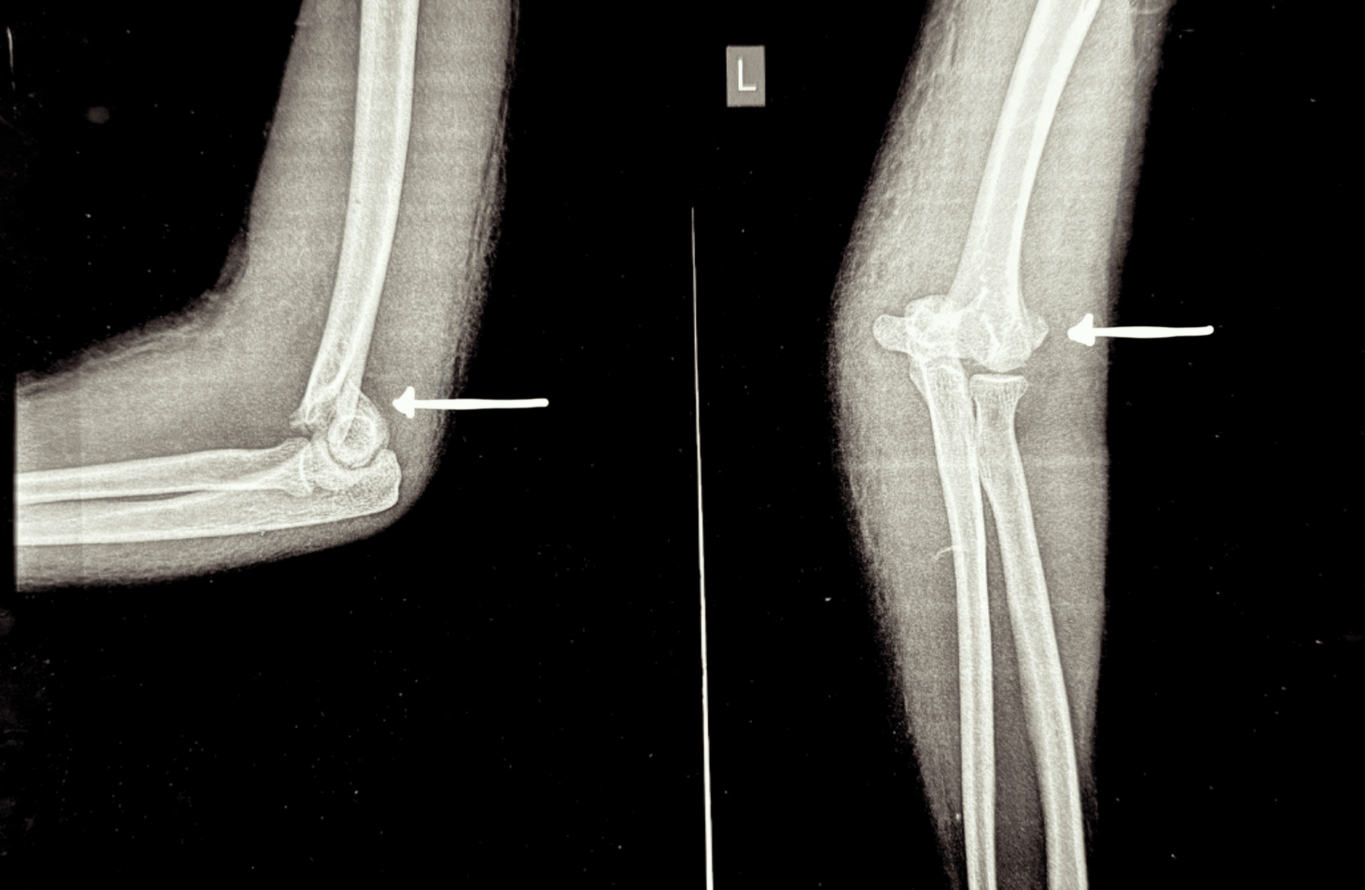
Anteroposterior and lateral radiographs of the left elbow showing a distal humerus fracture
